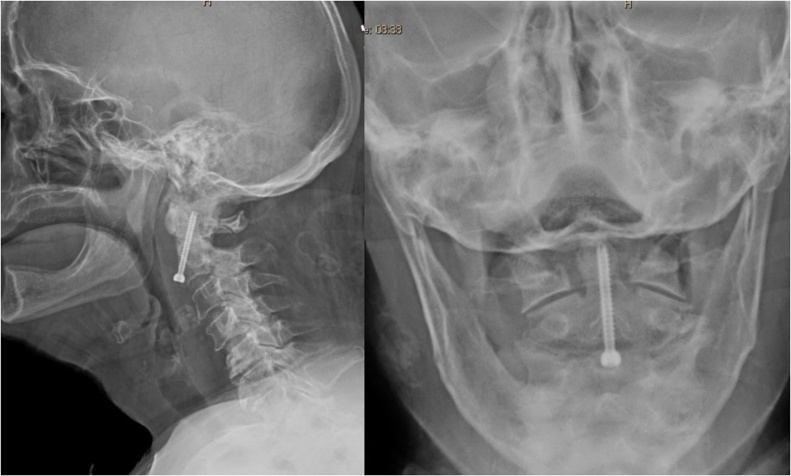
Final X-rays (lateral and open mouth views) showing maintained reduction of the fixation of the fracture.
